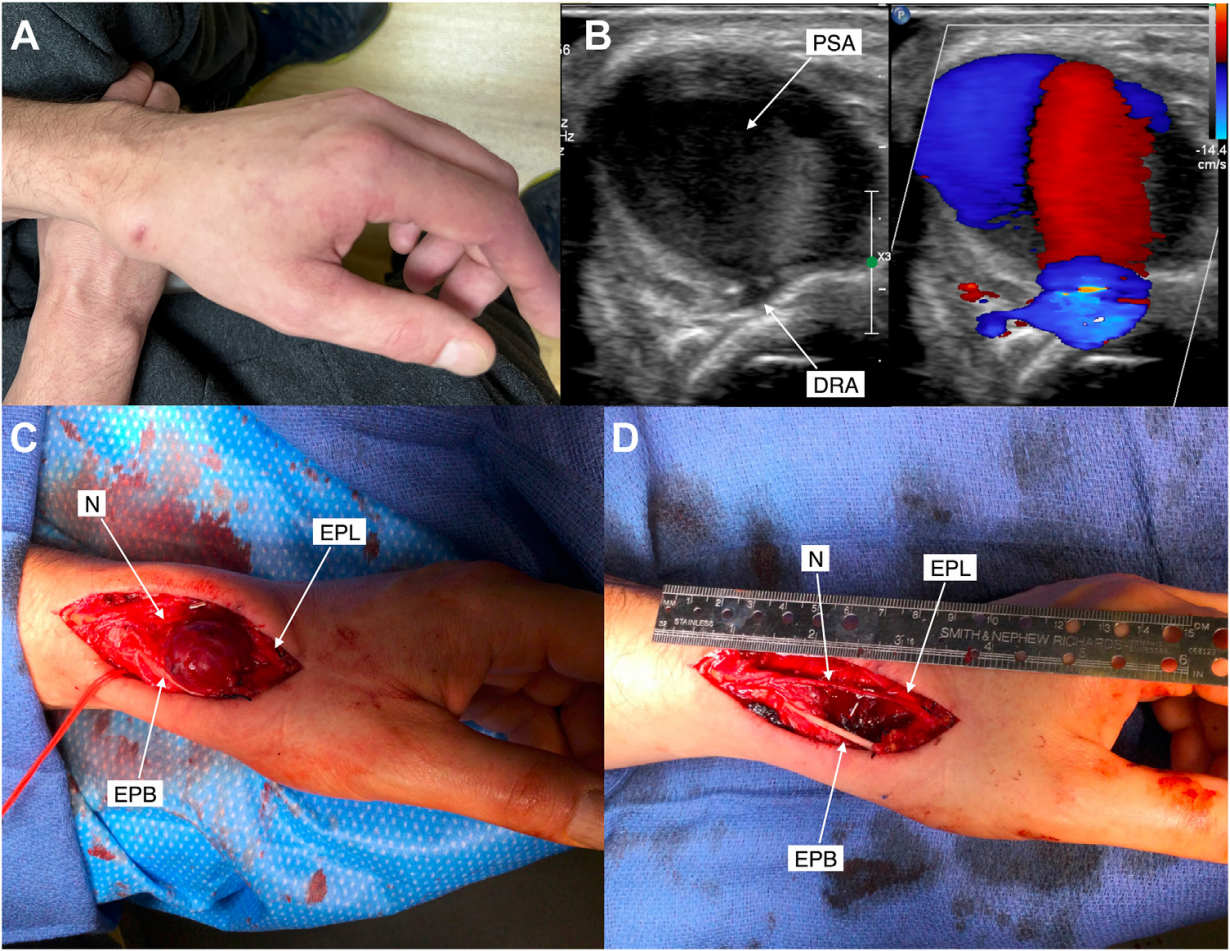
Distal radial artery pseudoaneurysm. (A) Clinical appearance of the pseudoaneurysm at the left distal radial access site 4 weeks after percutaneous coronary intervention. (B) Duplex and Doppler color flow ultrasound of the pseudoaneurysm and distal radial artery. Operative appearance of the pseudoaneurysm (C) on exposure and (D) after resection, showing a dorsal digital branch of the radial nerve, tendons of the extensor pollicis longus, and the extensor pollicis brevis. DRA, distal radial artery; EPB, extensor pollicis brevis; EPL, extensor pollicis longus; N, nerve; PSA, pseudoaneurysm.
